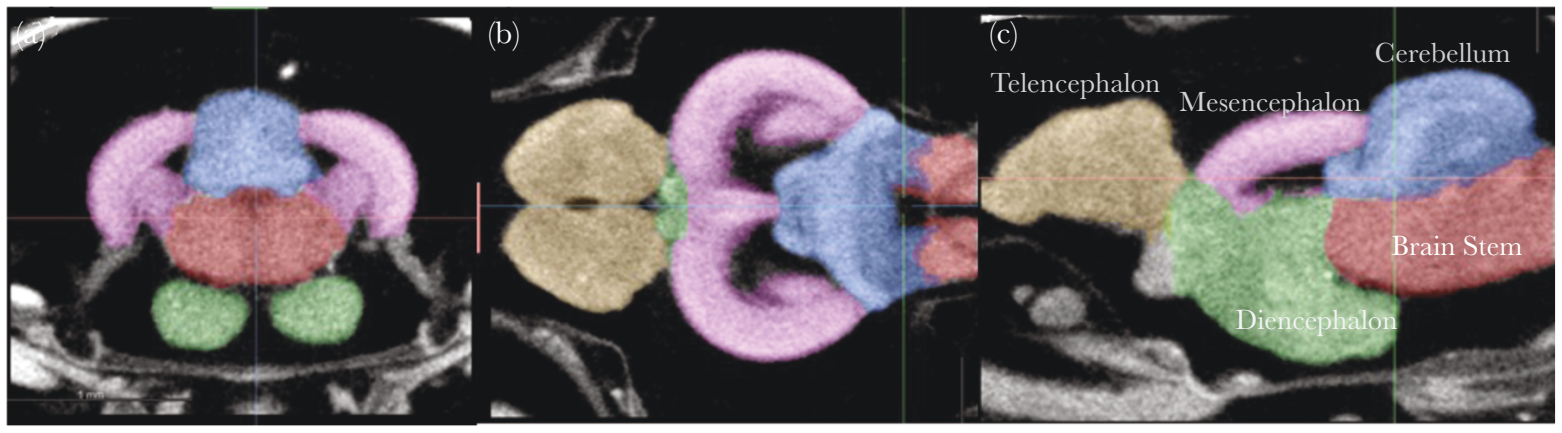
Segmented fish brain X-ray images. (a) Transversal, (b) coronal, and (c) sagittal planes of a brain scan. The major five brain parts were segmented and displayed in different colours: yellow–telencephalon, purple–mesencephalon, green–diencephalon, blue–cerebellum, and red–brain stem.
